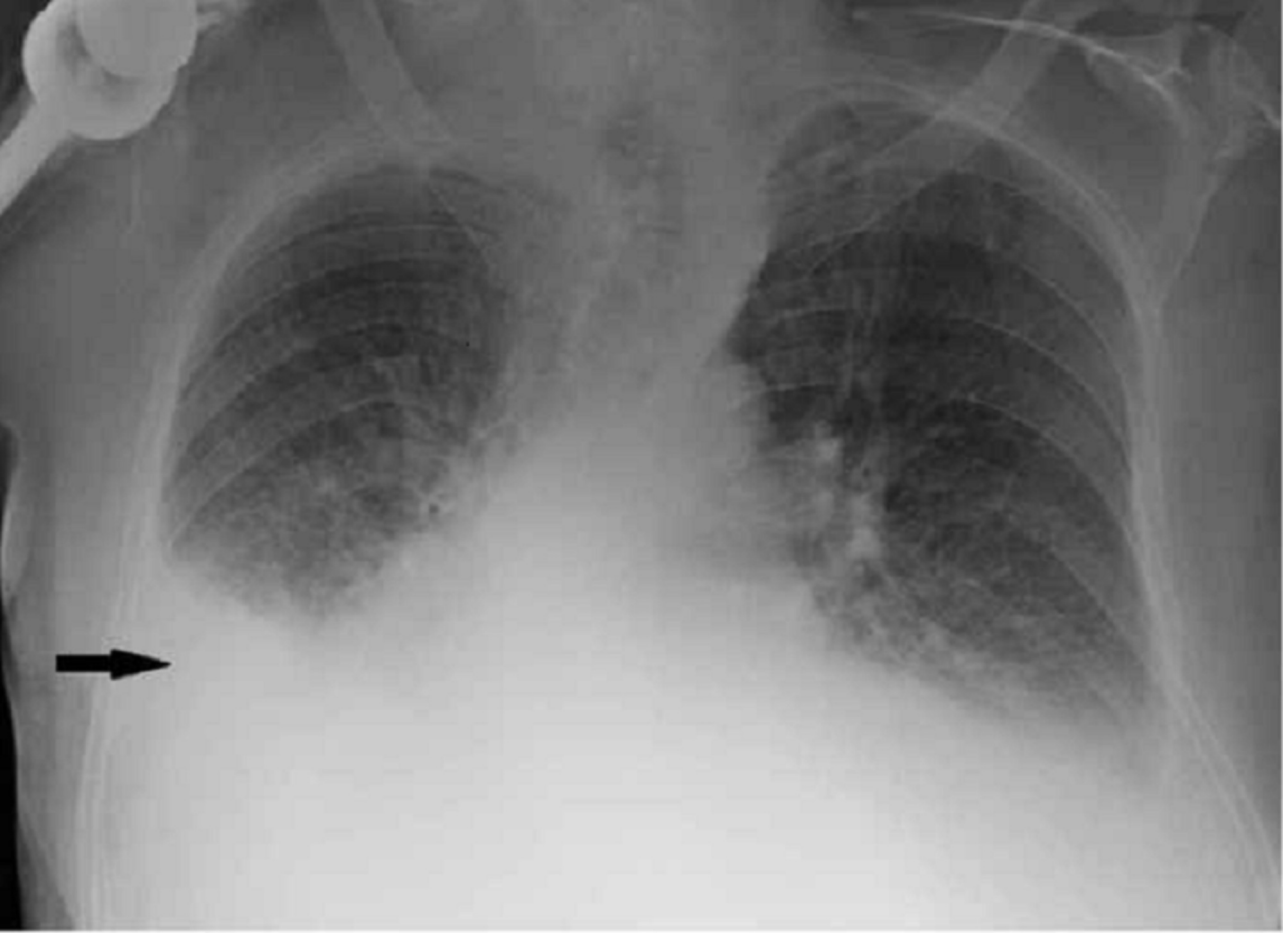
Posteroanterior radiograph showing moderate size right sided pleural effusion (arrow)
